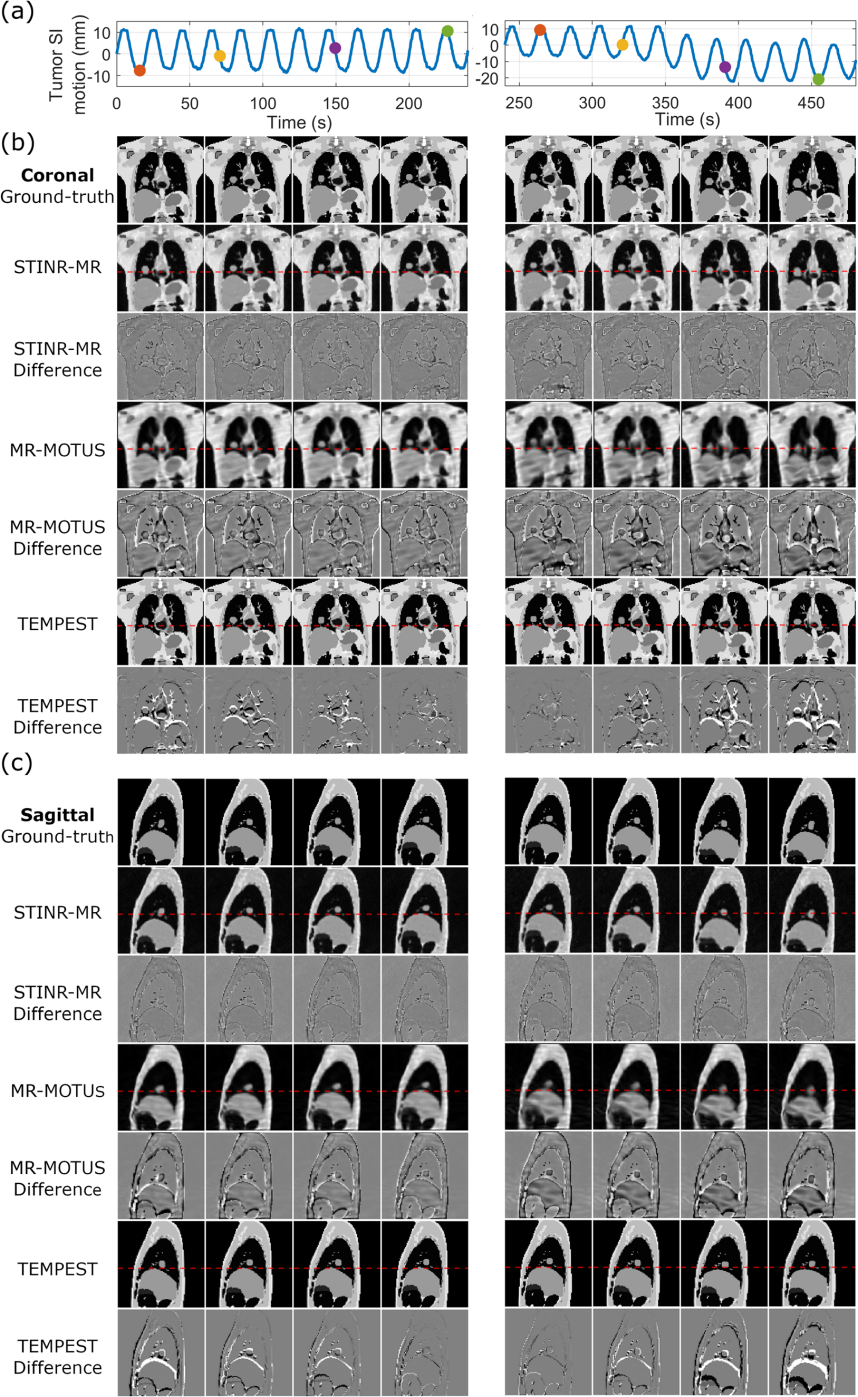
Comparison between reconstructed cine-MR images by STINR-MR (offline PCA), MR-MOTUS, and TEMPEST for the XCAT S1 (left column) and S2 (right column) motion scenarios in the (b)coronal and (c) sagittal views. The corresponding difference images between the ‘ground-truth’ and the reconstructions were also shown. The top panels (a) in each view depict the STINR-MR-solved lung tumor motion trajectories in the SI direction, and the dots indicate the motion states selected for plotting.
